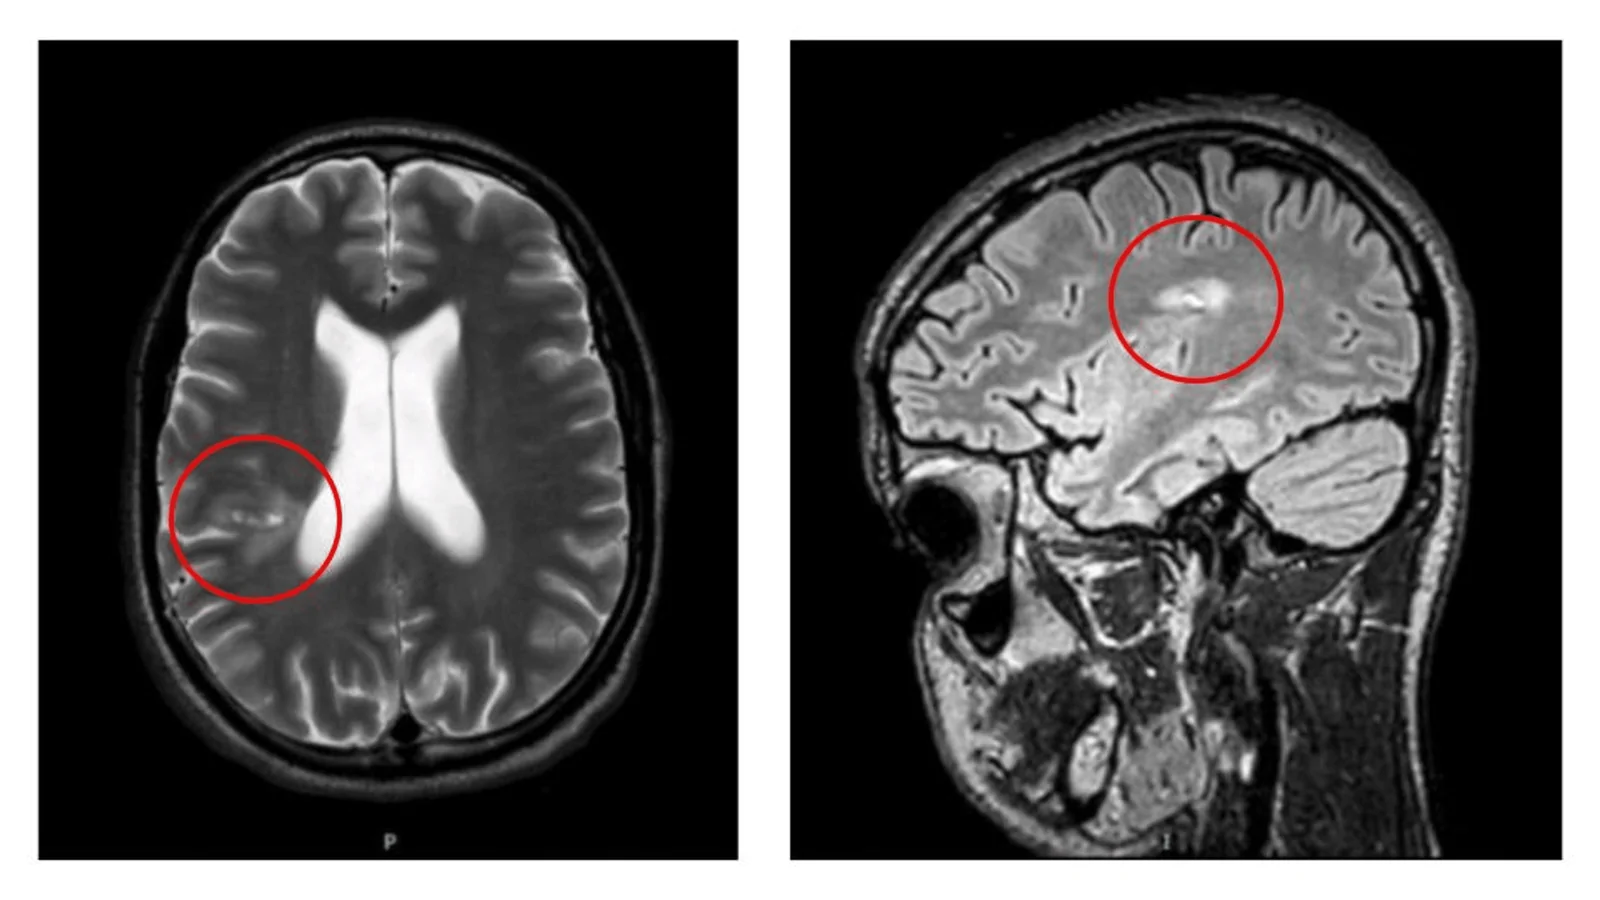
MRI brain with and without contrast after four weeks of antimicrobial therapy
